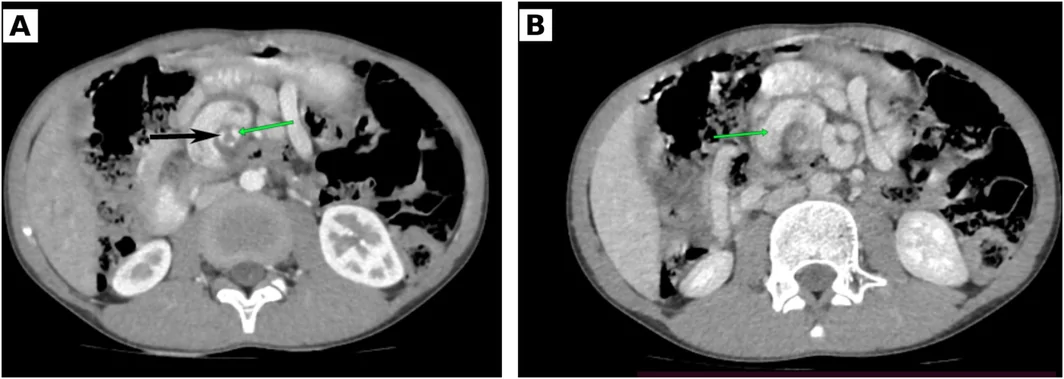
Axial contrast‐enhanced CT of the abdomen. (A) Reversal of the normal SMA–SMV relationship, with the superior mesenteric artery (SMA) to the right (black arrow) and the superior mesenteric vein (SMV) to the left (green arrow). (B) Whirling of the SMV and mesenteric fat around the SMA axis, producing the characteristic whirlpool sign.
